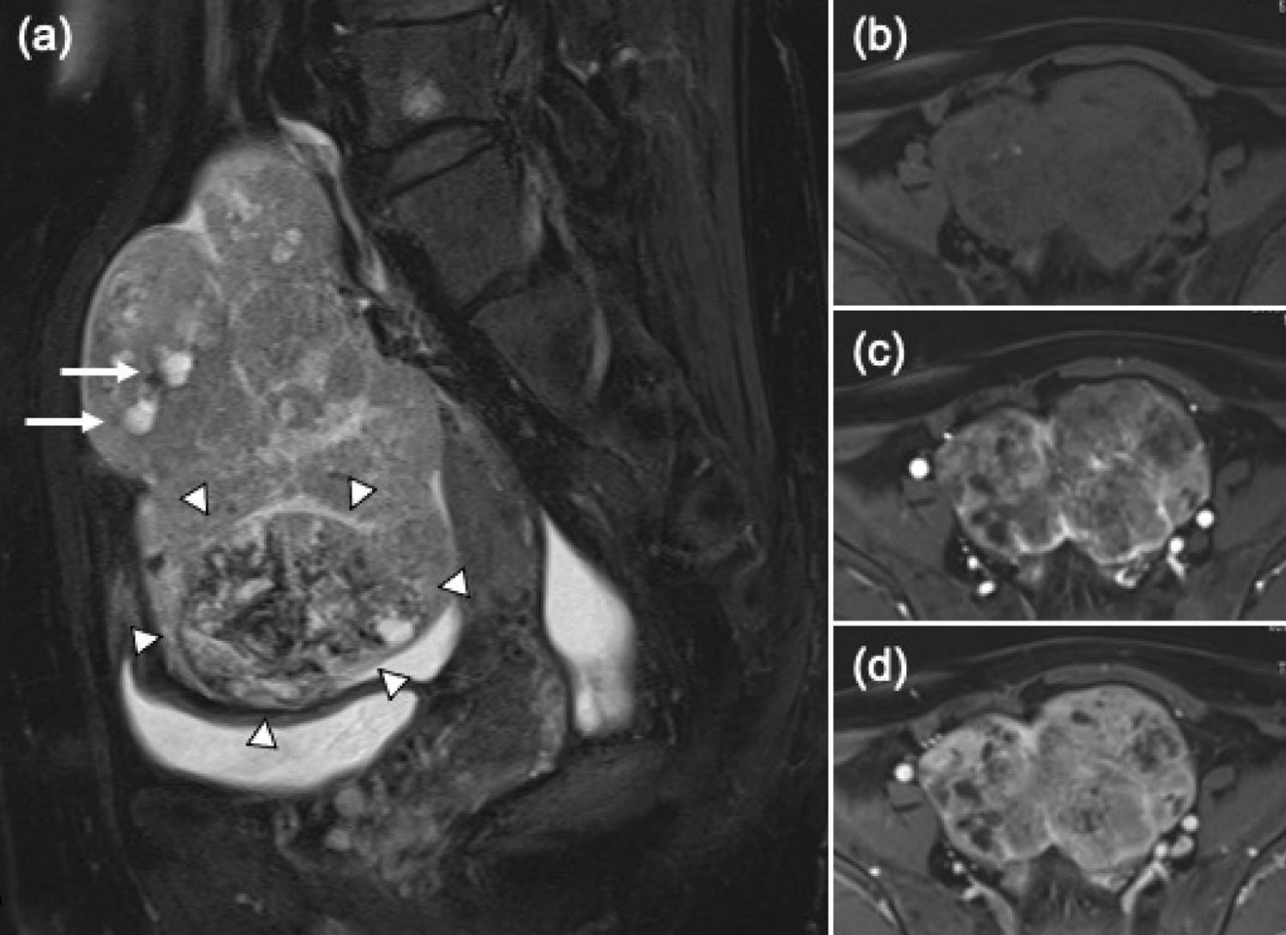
Magnetic resonance images of pelvis. a T2-weighted sagittal image showing a 11-cm tumor including cystic (arrows) and necrotic lesions with hemorrhage (arrowheads). b–d Dynamic contrast-enhanced magnetic resonance images showing tumor hypervascularity (b plain phase; c early phase; d delayed phase)
